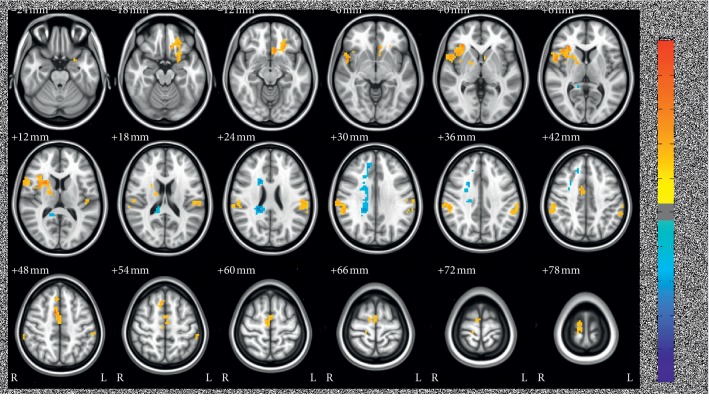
Group analysis results of activation in response to ultrasound stimulation at ST 36.
